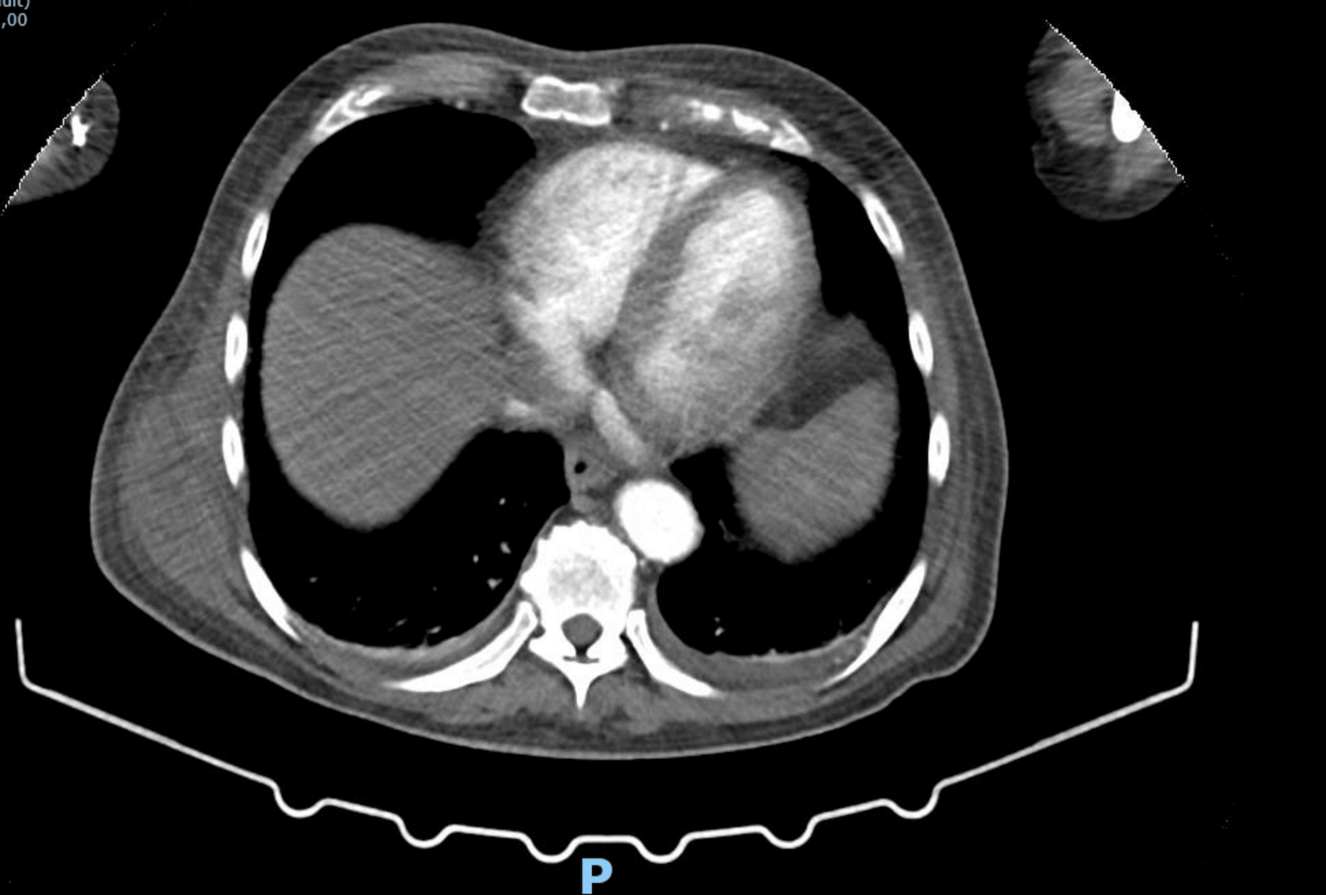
CT scan revealing abscess of the right latissimus dorsi muscle CT scan revealing an heterogenous area on the lateral aspect of the right latissimus dorsi muscle, located in the lateral region of the lower third of the right hemithorax, measuring approximately 63 x 35 mm with peripheral enhancement after intravenous contrast administration (abscess or infected hematoma) without evidence of active bleeding.
